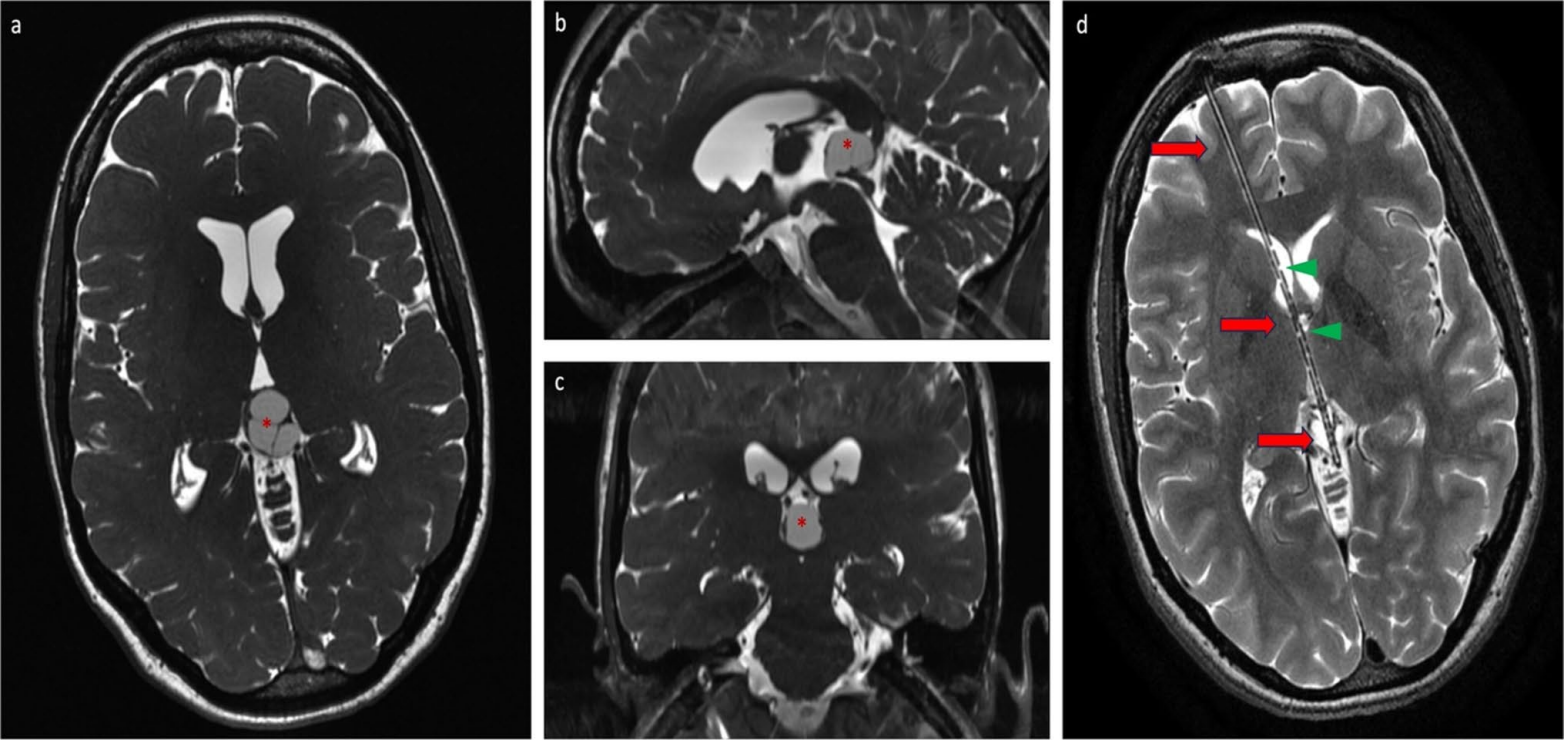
Pre- (a-c) and postoperative (d) MRI imaging of a pineal cyst (red *) and consecutive hydrocephalus occlusus drained through an internal shunt. The course of the catheter is marked by the red arrow. Extra perforations in the catheter to achieve optimal up- and downstream drainage (green arrow head) were added manually. Additionally, a normalized ventricular system can be seen postoperatively
